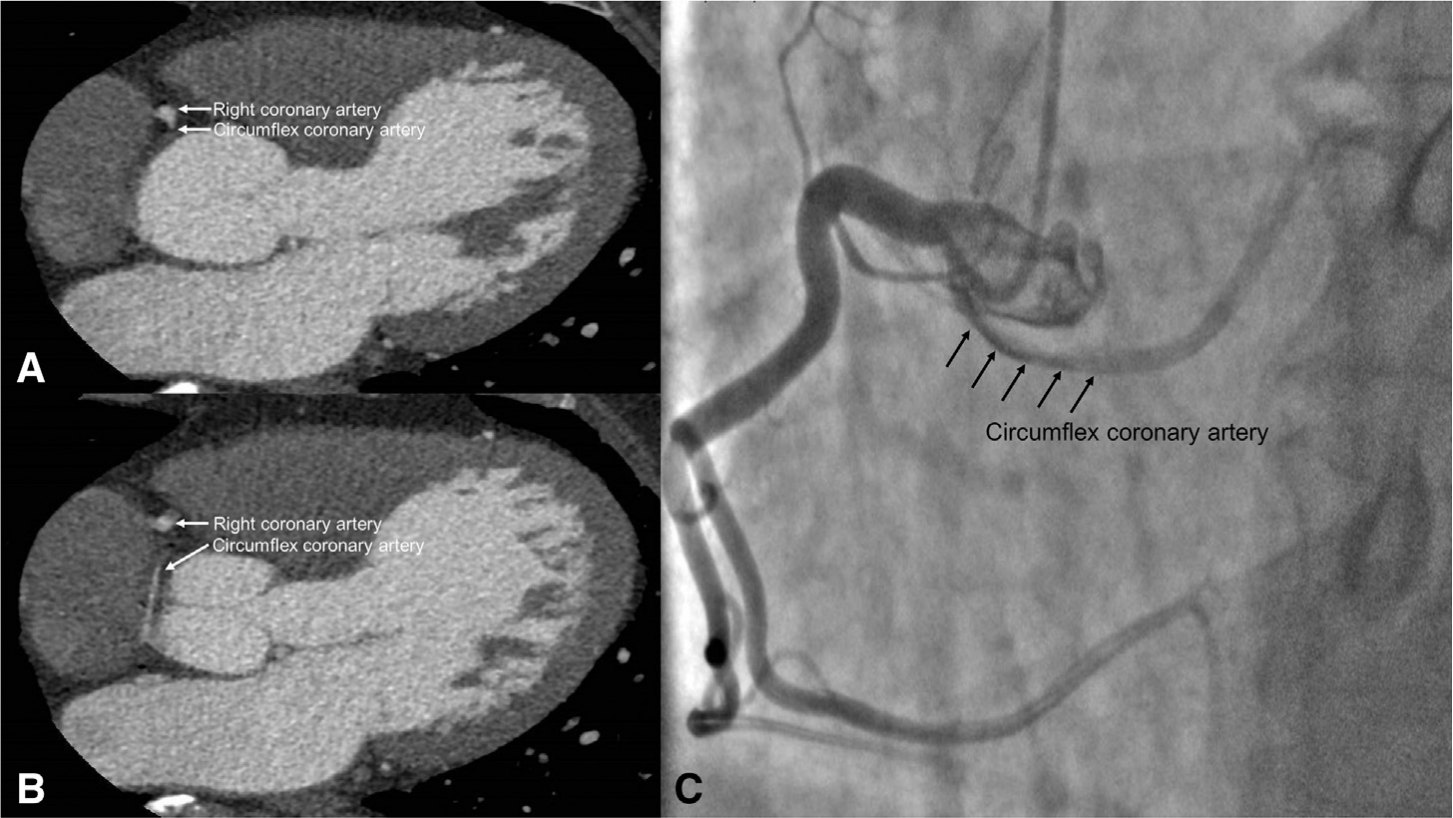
A, Contrast computed tomography showing the right coronary artery and a retroaortic anomalous left circumflex artery originating from the right coronary artery. B, The circumflex coronary artery courses in close proximity and inferior to the annulus of the noncoronary sinus. The diameter of aortic root measures 53 mm. No calcification is observed in the aortic valve. C, Coronary angiography demonstrating an anomalous left circumflex artery originating from the right coronary artery (*arrows*). A nonobstructive stenosis in the left circumflex artery is noted. The right coronary is dominant and free of obstructive coronary artery disease.
